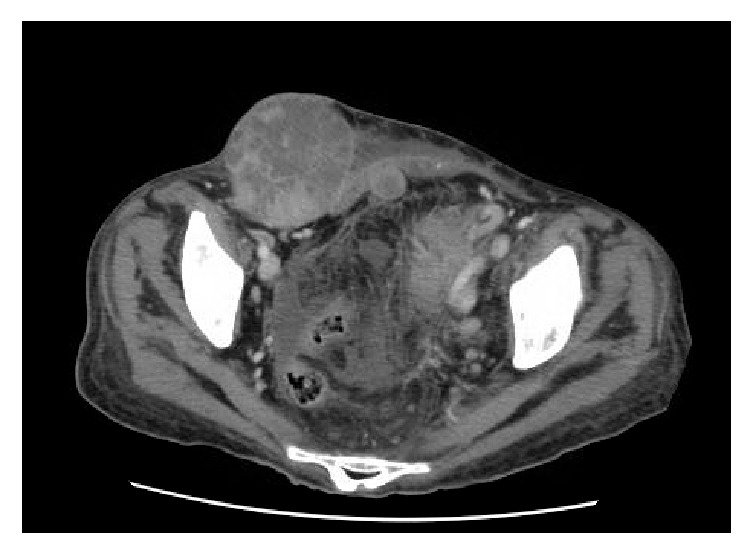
Axial CT scan showing heterogeneous 14 × 12 cm pelvic-abdominal mass between uterus and rectum, most likely originating from the left ovary and inseparable from the posterior uterus.
